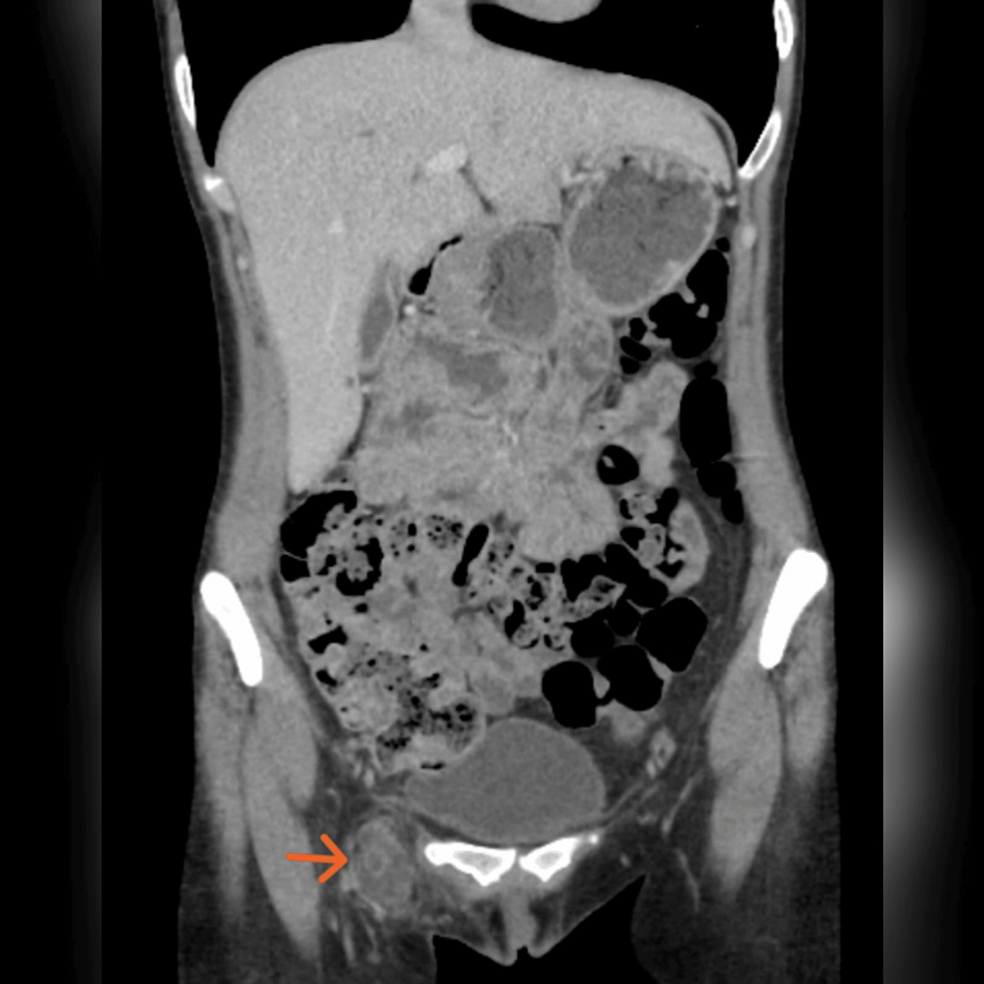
CT abdomen and pelvis showing a cystic lesion in the right femoral region (orange arrow) with no femoral or inguinal hernia
